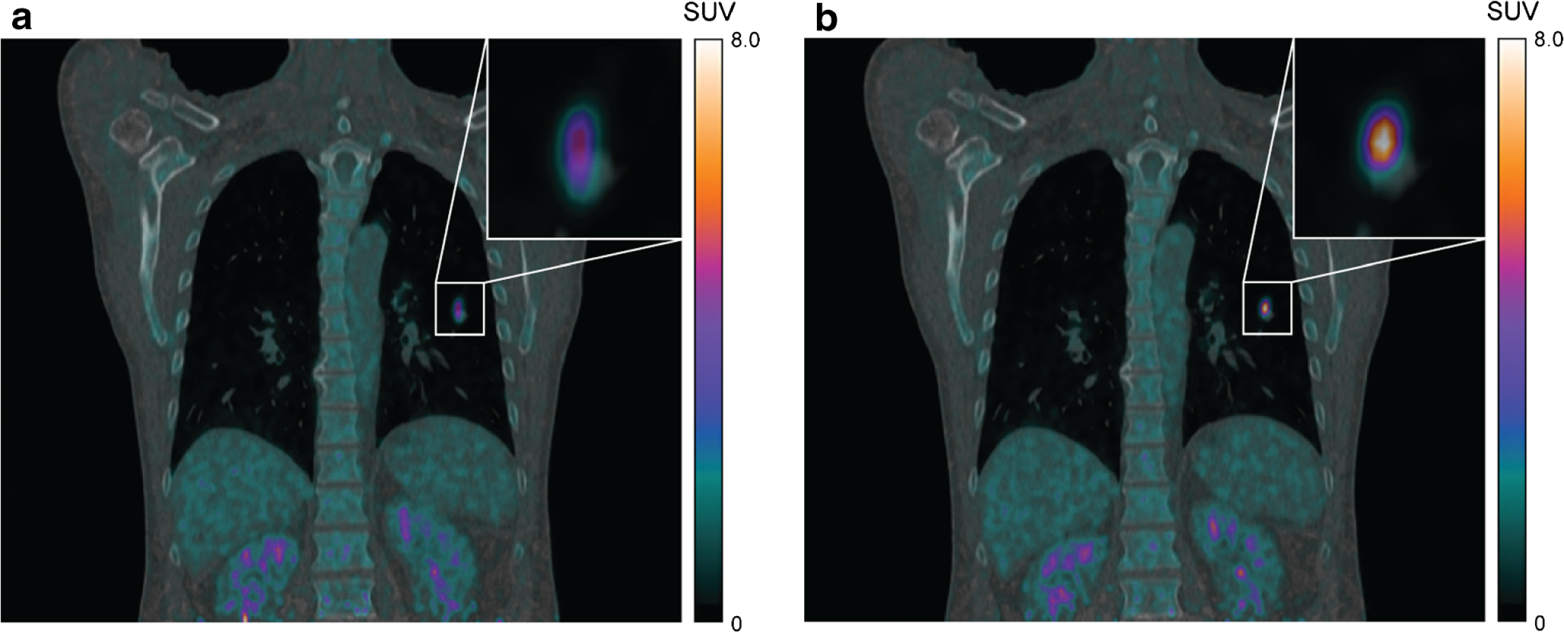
^18^F–FDG PET/CT images (mCT, Siemens) of a patient with a non-small cell lung cancer lesion in the left lower lobe. (a) Non-gated and (b) an essentially motion-free image (HD•Chest). Both PET images have been reconstructed with a matrix size of 400 × 400, pixel size of 2 × 2 mm^2^, with PSF modeling and TOF. For the non-gated images, the first 35% (126 s) of the acquired data was used for image reconstruction, resulting in an equal number of acquired true coincidences as the gated image. There is a considerable increase in SUV_mean_ of 70% and a decrease in volume of 80%. Images have been reproduced from [46]
